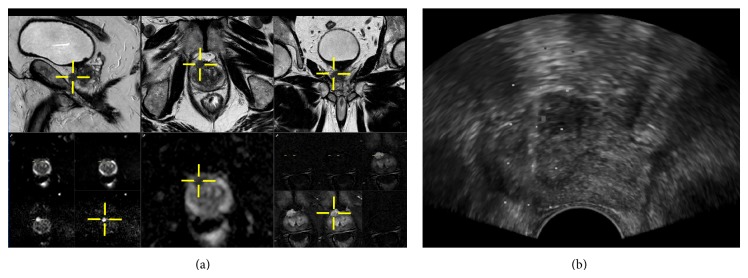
Mp-MRI targeted TRUS-G biopsy of clinically significant prostate cancer. 66-year-old patient with a PSA of 8 ng/mL. Prebiopsy mp-MRI (a) shows a 9 mm low T2 nodule (image (a); upper row), with high retriction of water diffusion and hypervascularization (image (a); lower row) in the anterior horn of the right apical PZ, ahead of the posterior 18 mm of gland sampled by SB cores. A TB was performed with knowledge of this information. It diagnosed a CSPCa (4 out of 4 positive TB; no SB was positive). Image (b) shows the trace of the needle biopsy gun inside the nodule. This lesion was aimed with visual registration, thanks to its zonal anatomy (ahead of the anterior TZ, at the edge of the anterior prostate surface), its size, and the presence of a small cyst in the right TZ (not visible on image (b), but used to locate the craniocaudal location of the lesion at time of biopsy).
